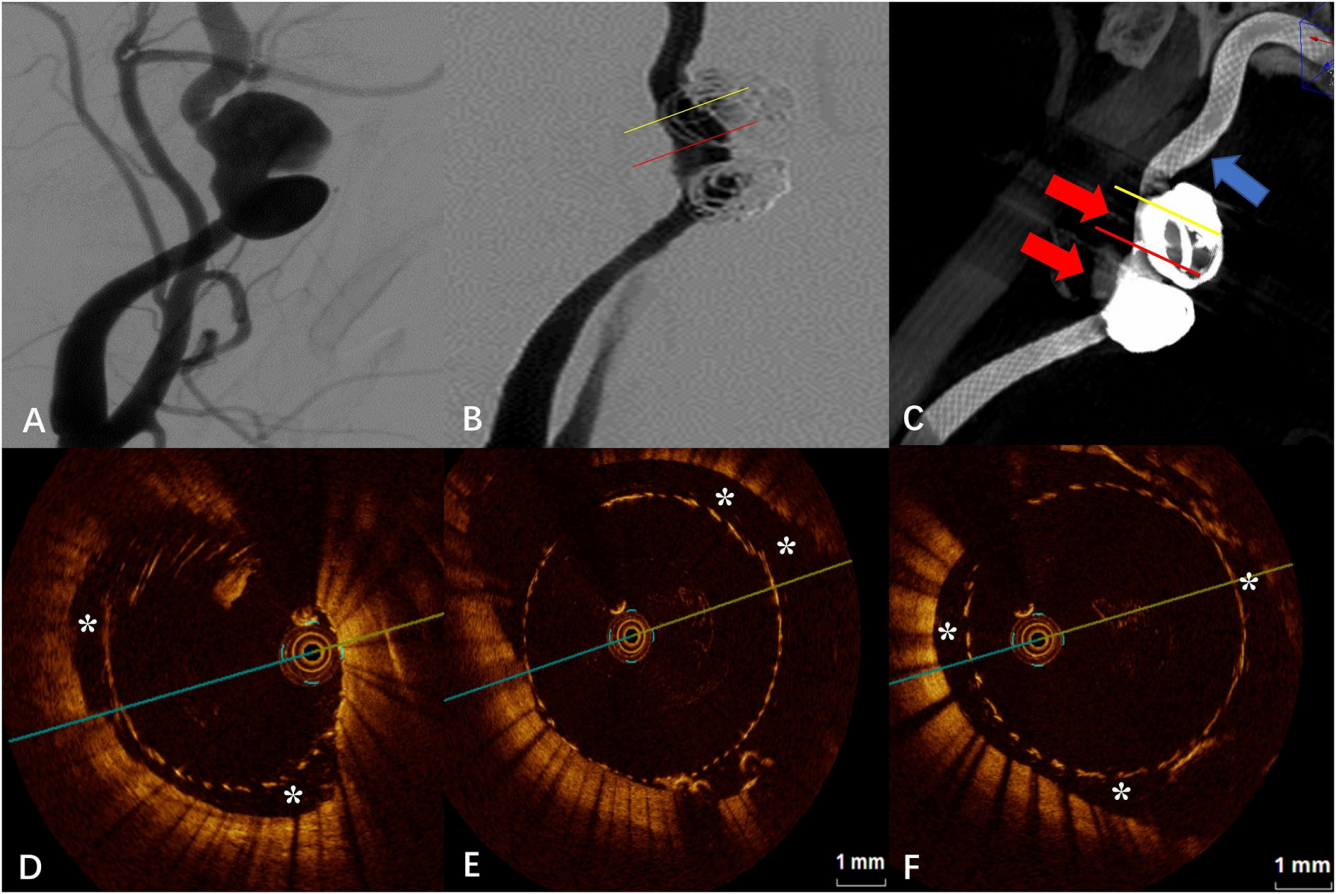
(A) Digital subtraction angiography (DSA) before the flow-diverter placement. (B) Digital subtraction angiography (DSA) just after the flow-diverter placement. (C) Vaso CT images. Localized malapposition in distal parent artery (blue arrow) and proximal parent artery with good apposition of the FD to the wall. Artifacts near the aneurysm neck that disturb the evaluation of apposition by Vaso CT (red arrows). (D) OCT image. Corresponding to localized malapposition [blue arrow in (C)] confirmed by Vaso CT (*). (E) OCT image. The corresponding yellow line in (B,C). Eccentric communication malapposition near the aneurysm neck (*). (F) OCT image. The corresponding red line in (B,C). Circumferential communication malapposition at the aneurysm (*).
